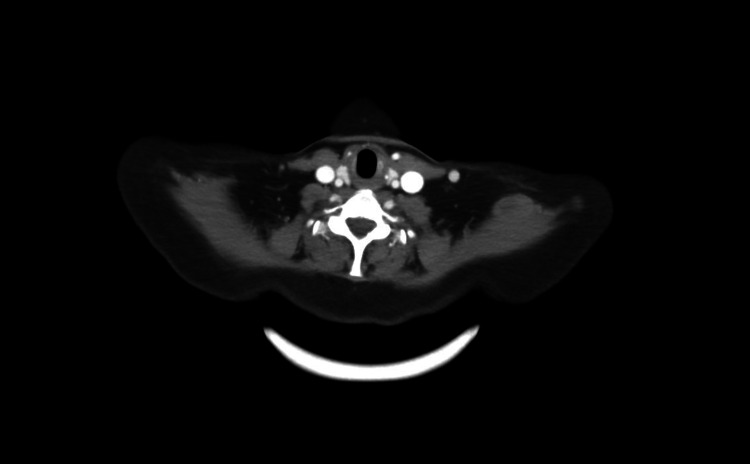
CT of chest/abdomen/pelvis with contrast showing disease response with the index lesion at the left trapezius decreased in size to 3.3 x 1.9 cms
